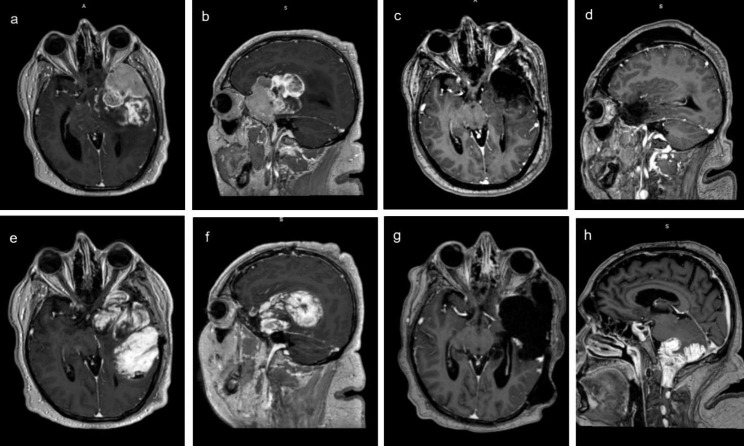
Radiological features. A voluminous tumour in the left temporal pole, extending superiorly to fronto-basal and fronto-lateral regions, deeply to the insula and posteriorly till the supramarginal gyrus was discovered (a, b). The tumour was initially interpreted as atypical meningioma with peripheral intratumoral degenerative cysts. The tumour was completely resected with no signs of residue; brain tissue’s compression resolved (c, d). A two months post-surgery MRI control demonstrated a huge left fronto-temporal local tumoral recurrence (e, f). Three months MRI control after a second intervention showed only a small local recurrence on the posterior margin of the surgical cavity but a diffuse leptomeningeal tumoral spread on the inferior surface of cerebellum, around the brainstem and around the spinal cord (g, h)
